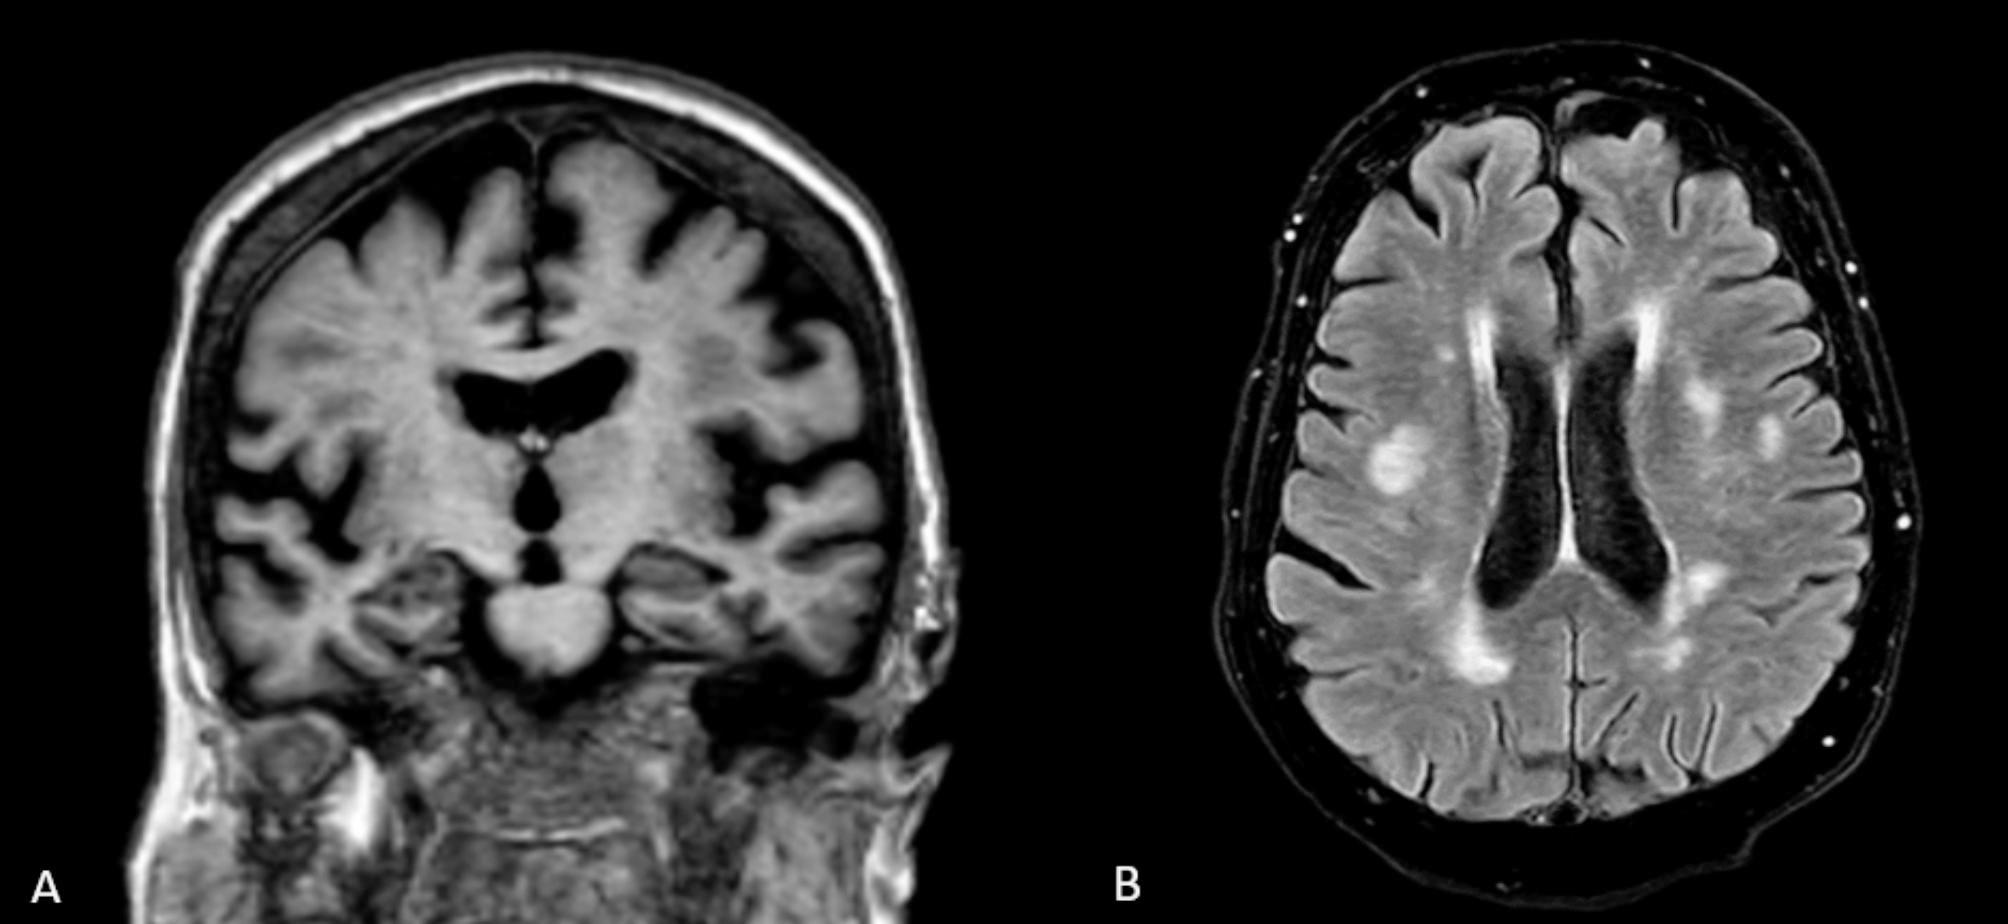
Non-contrast MRI of the brain on day 65 of admission. (A) Coronal view with age-related parenchymal changes without focal hippocampal atrophy. (B) Axial view showing mild chronic small vessel ischemic changes in the cerebral white matter
